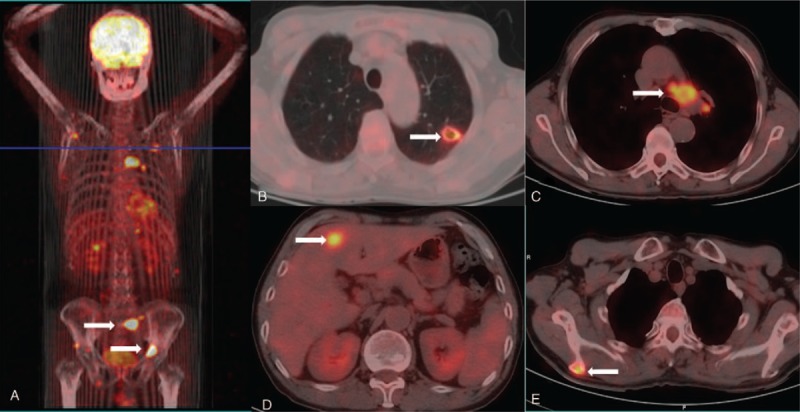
Positron emission tomography-computed tomography of the patient showed a small pulmonary cystic lesion, multiple hepatic masses, intramuscular and osteolytic damages (arrow) on June 6^th^, 2016, with abnormal uptake of Fluorine-18-fluorodeoxyglucose.
